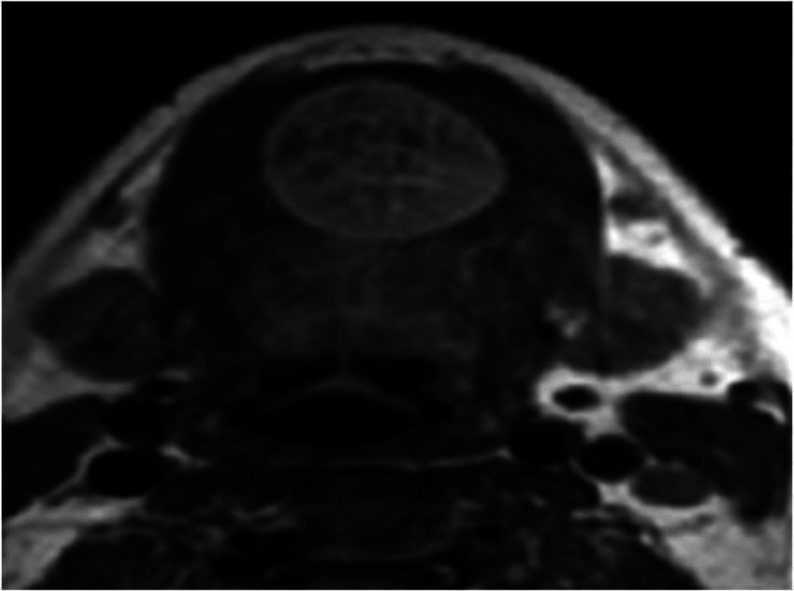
MRI of the tongue revealed the presence of a well-circumscribed nodule on the right anterior tongue, homogeneously and homo-geneously hyperintense on T2WI image
